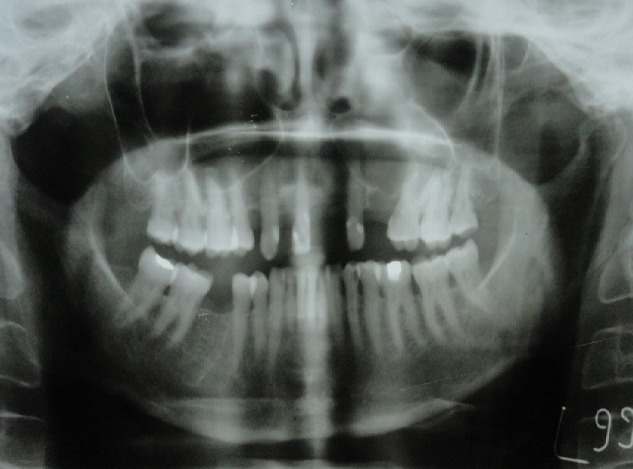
Panoramic radiograph showing an oval-shaped unilocular radiolucency with thin corticated borders extending from 23 to 26 regions
